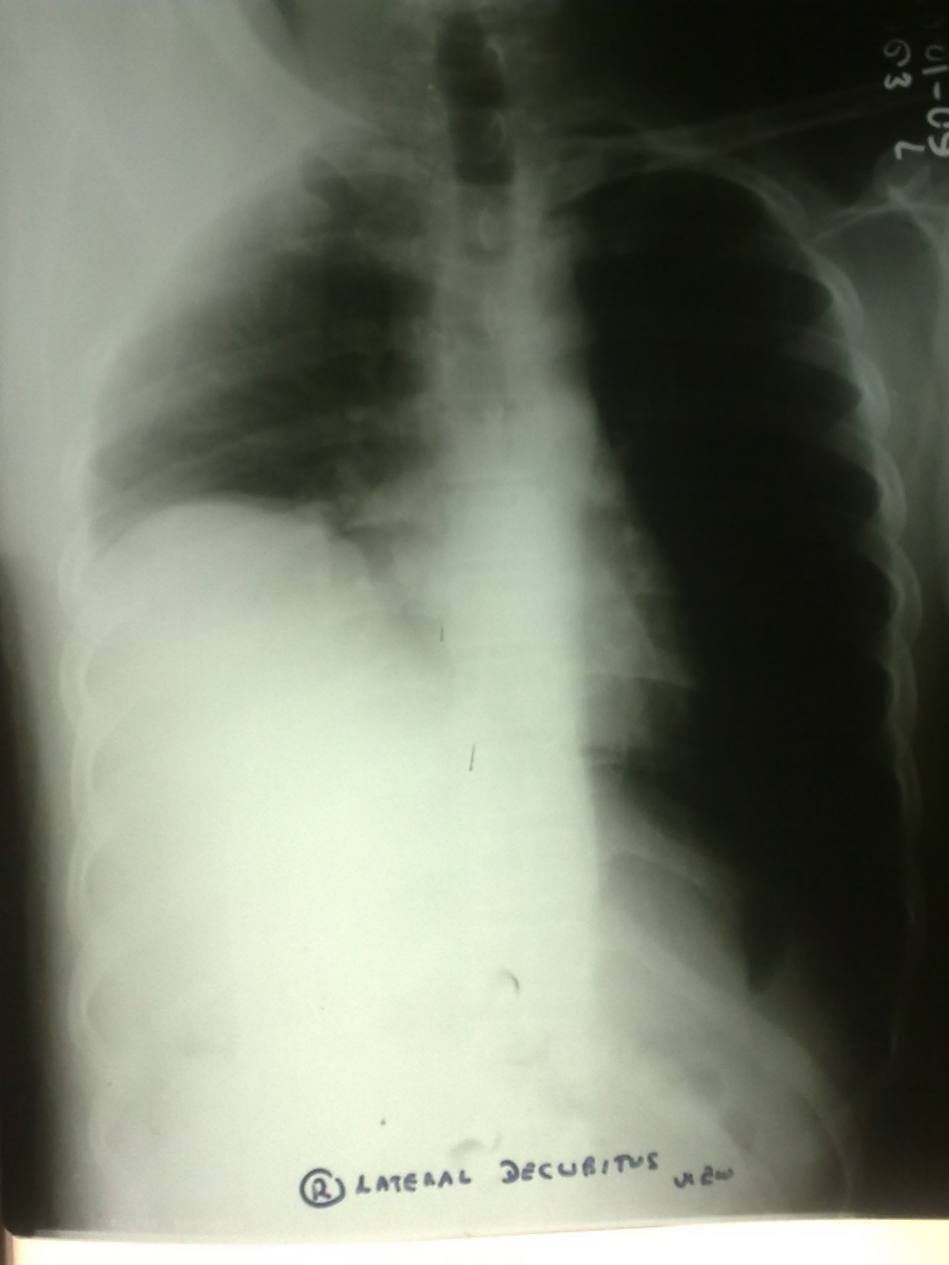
Chest X-Ray one month after initial discharge.
